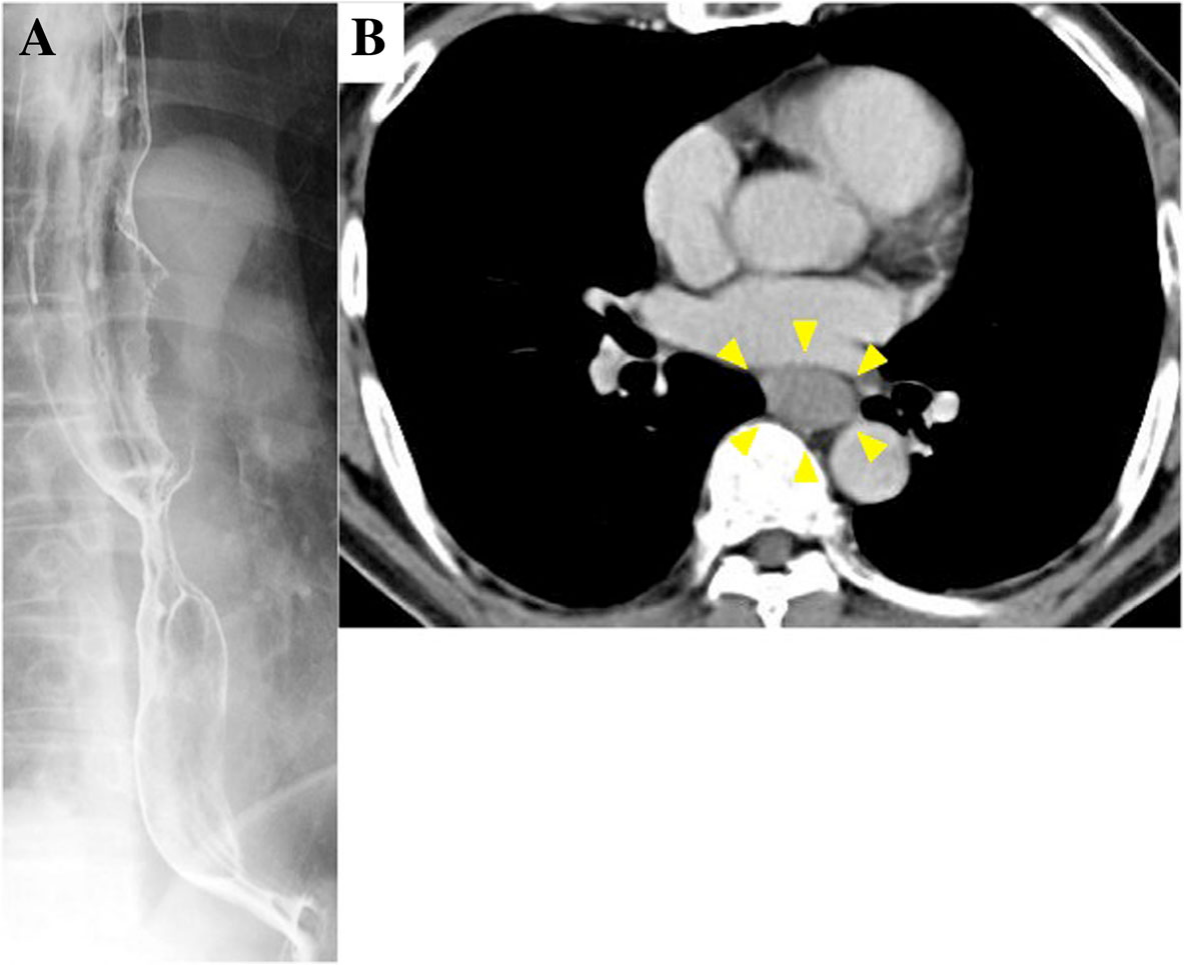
Before chemoradiotherapy. a Upper gastrointestinal series revealed subcircumferential esophageal stenosis at the middle thoracic esophagus. b Enhanced computed tomography revealed a tumor (*arrowhead*) without invasion into the surrounding tissue located at the middle thoracic esophagus
